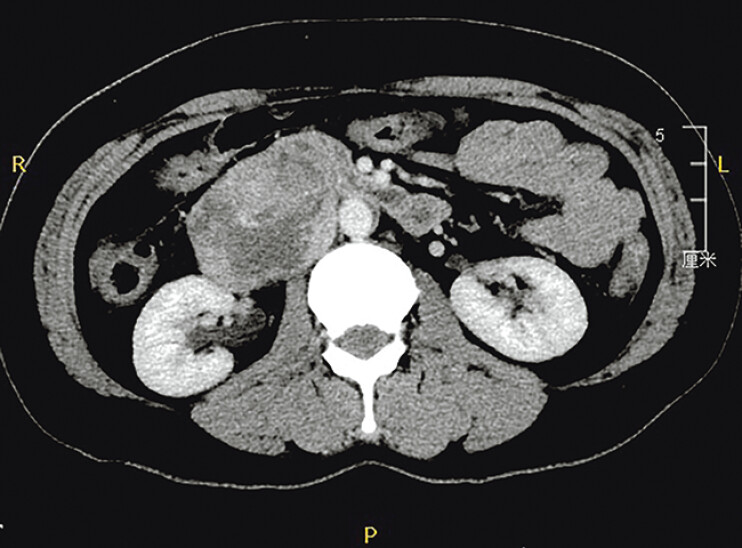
Contrast-enhanced computed tomography scan showed a mass of approximately 7 cm in diameter in the right retroperitoneum.
